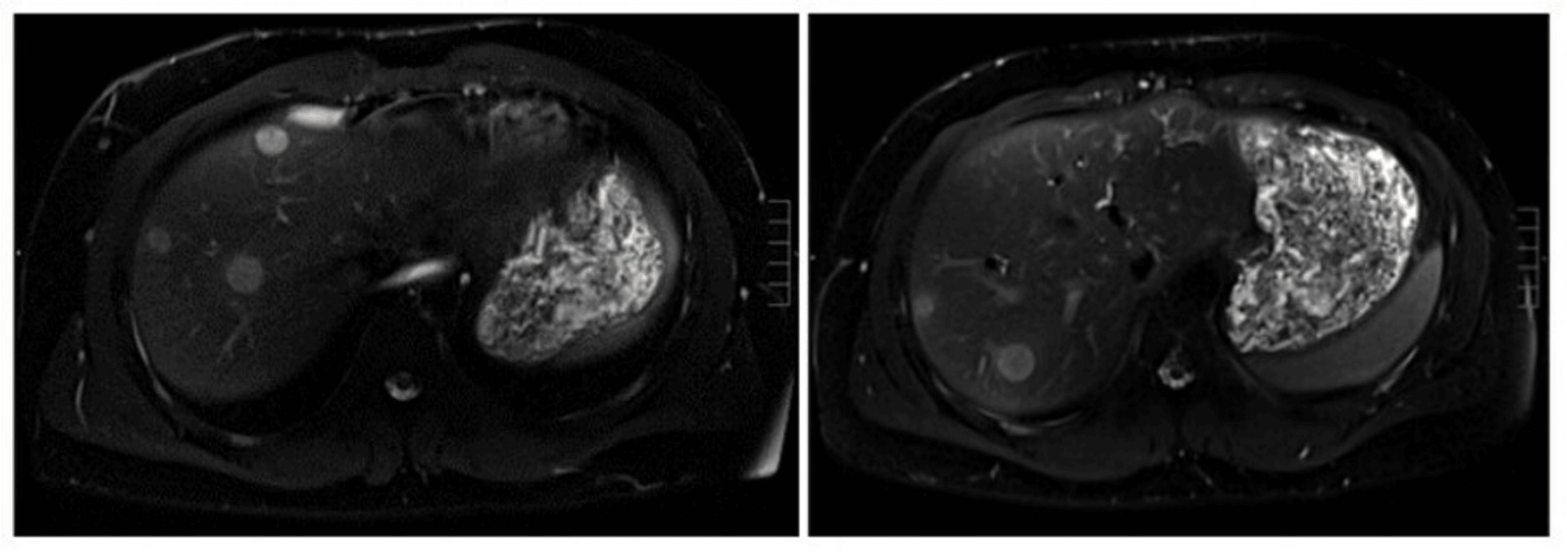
MRI abdomen T2 sequence showing multiple well-defined, rounded hyperintense liver lesions, concerning for metastatic disease. MRI, magnetic resonance imaging.
